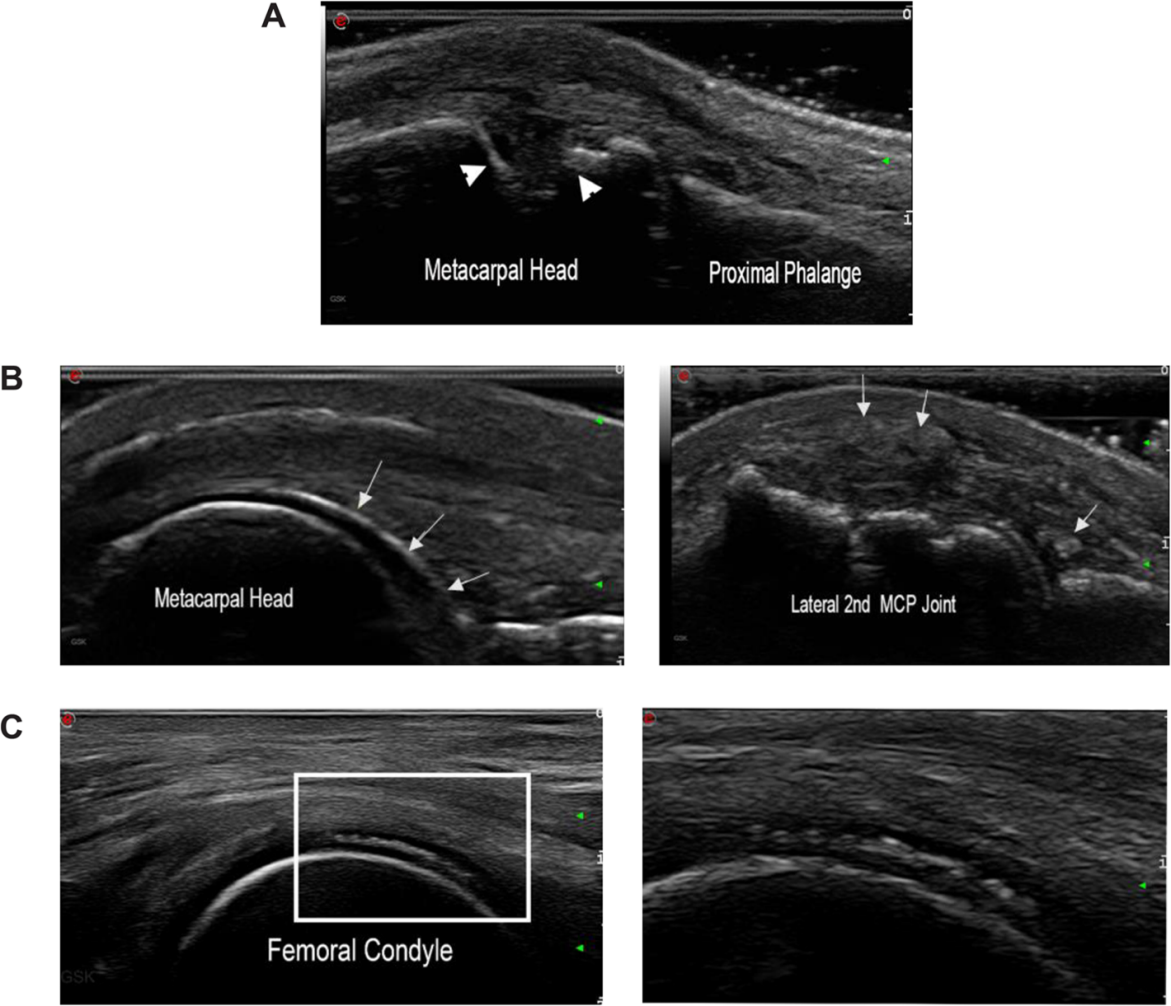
Ultrasound imaging of bone erosions and crystal deposits. a Transverse view of second metacarpophalangeal joint in a patient with rheumatoid arthritis; arrowheads denote bone erosion. b Left, chondrosynovial urate deposition at the second metacarpophalangeal joint (arrows); right, at the same joint, intra- and peri-articular tophaceous deposits seen as heterogeneous collections (arrows). c Left, calcium pyrophosphate crystal deposition seen sandwiched within the cartilage; right, magnified view of the white rectangular area on the left
